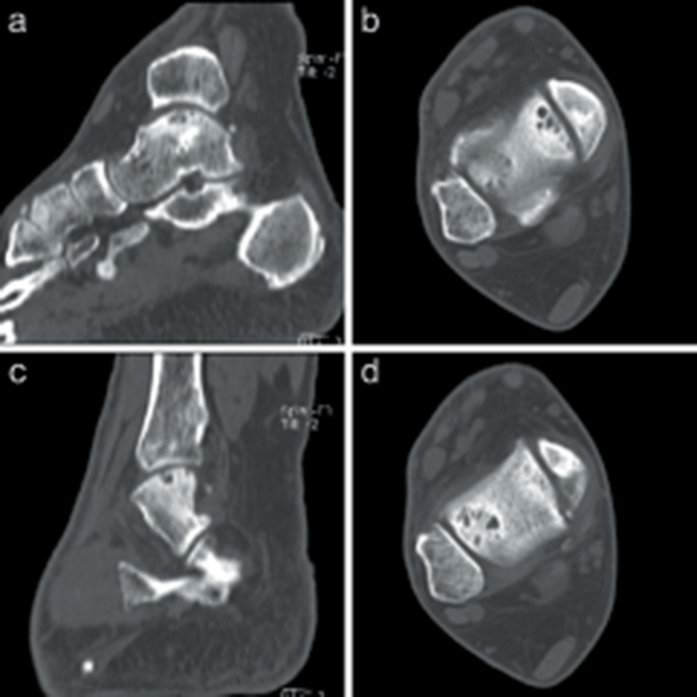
Preoperative computed tomography images. Preoperative (a) sagittal and (b) axial computed tomography images of OLT on medial talar dome. (c) Sagittal and (d) axial computed tomography images of OLT on lateral talar dome.
